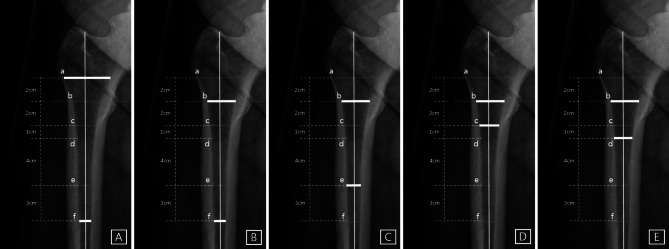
Radiographic parameters used to analyze proximal femoral morphology: (A) canal–flare index = a/f (B) canal–calcar ratio = f/b (C) modified morphological cortical index = b/e (D) LT-to-distal ratio-α = b/c (E) LT-to-distal ratio-β = b/d
